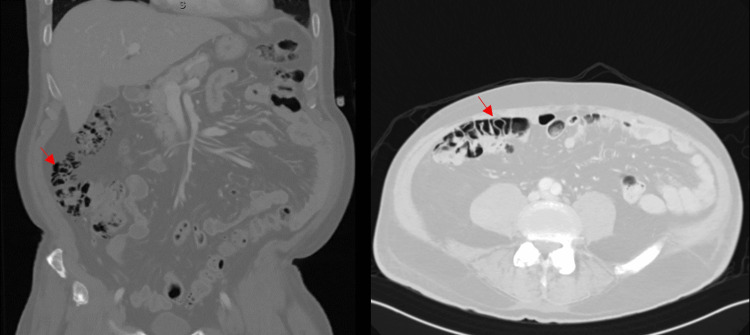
Left: coronal section with notable PI in the ascending colon (arrow). Right: cross-sectional abdominal CT with PI in the ascending colon (arrow).
